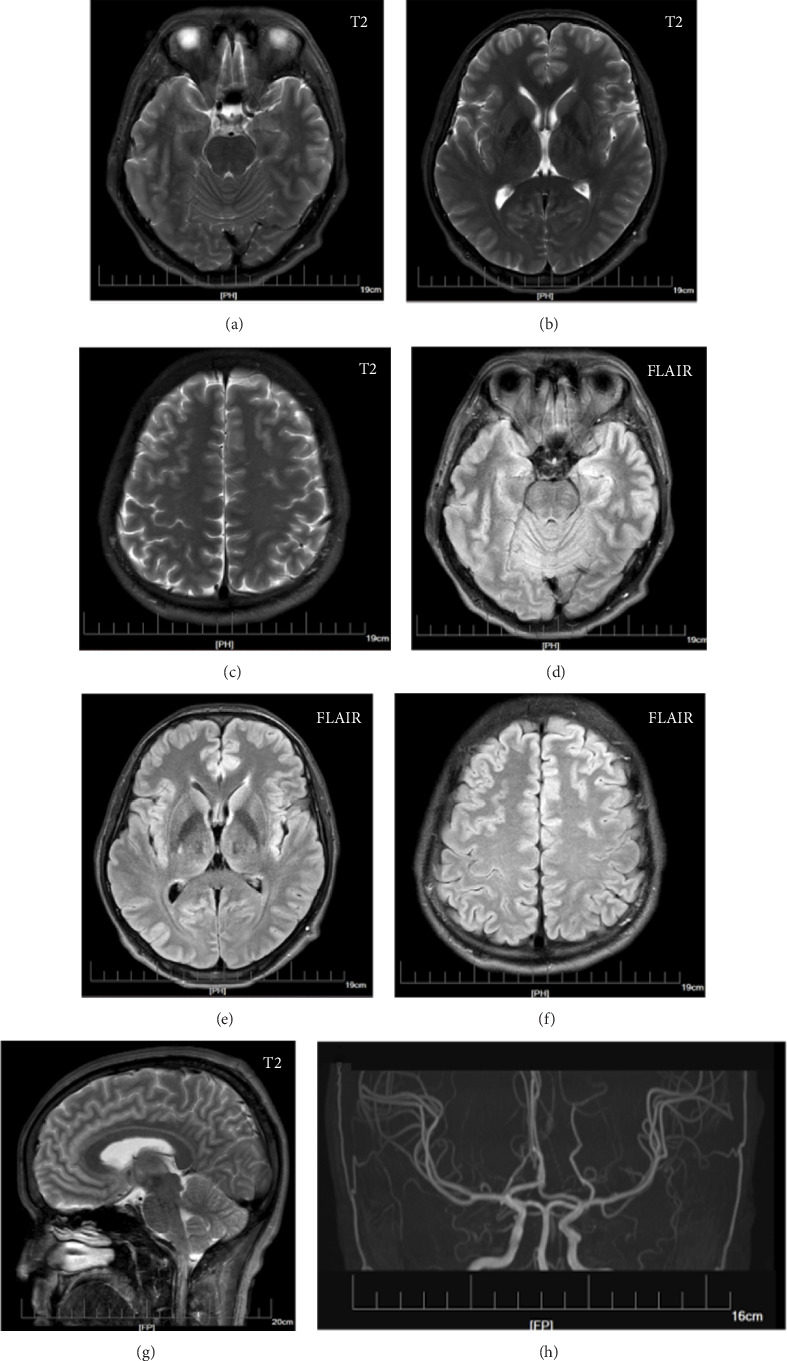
MRI and MRA images of a patient with macrocephaly. Axial T2WI (a–c), FLAIR (d–f) and sagittal T2WI (g) images of different patient cross-sections are shown. The cerebrovascular features were detected by MRA (h).
